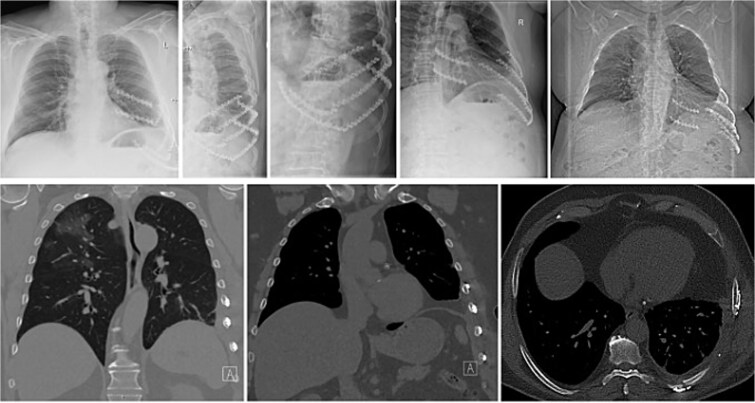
Postoperative X-ray and CT images. The X-ray (top row) shows the two rigid plates (green, solid, KLS Martin) bridging the non-union and bone grafting of the eighth and ninth rib in the posterior column. Additionally the costal margin along the rupture line is fixed with two plates in the cartilage tissue. Four plates (sixth–ninth rib) are applied to the anterolateral column of the sixth to ninth rib to prevent the fibre tape from cutting through the ribs. The bottom images show coronal and axial CT images with the reconstruction of the costal margin as well as the diaphragm and the non-union of the eighth and ninth rib posterior.
